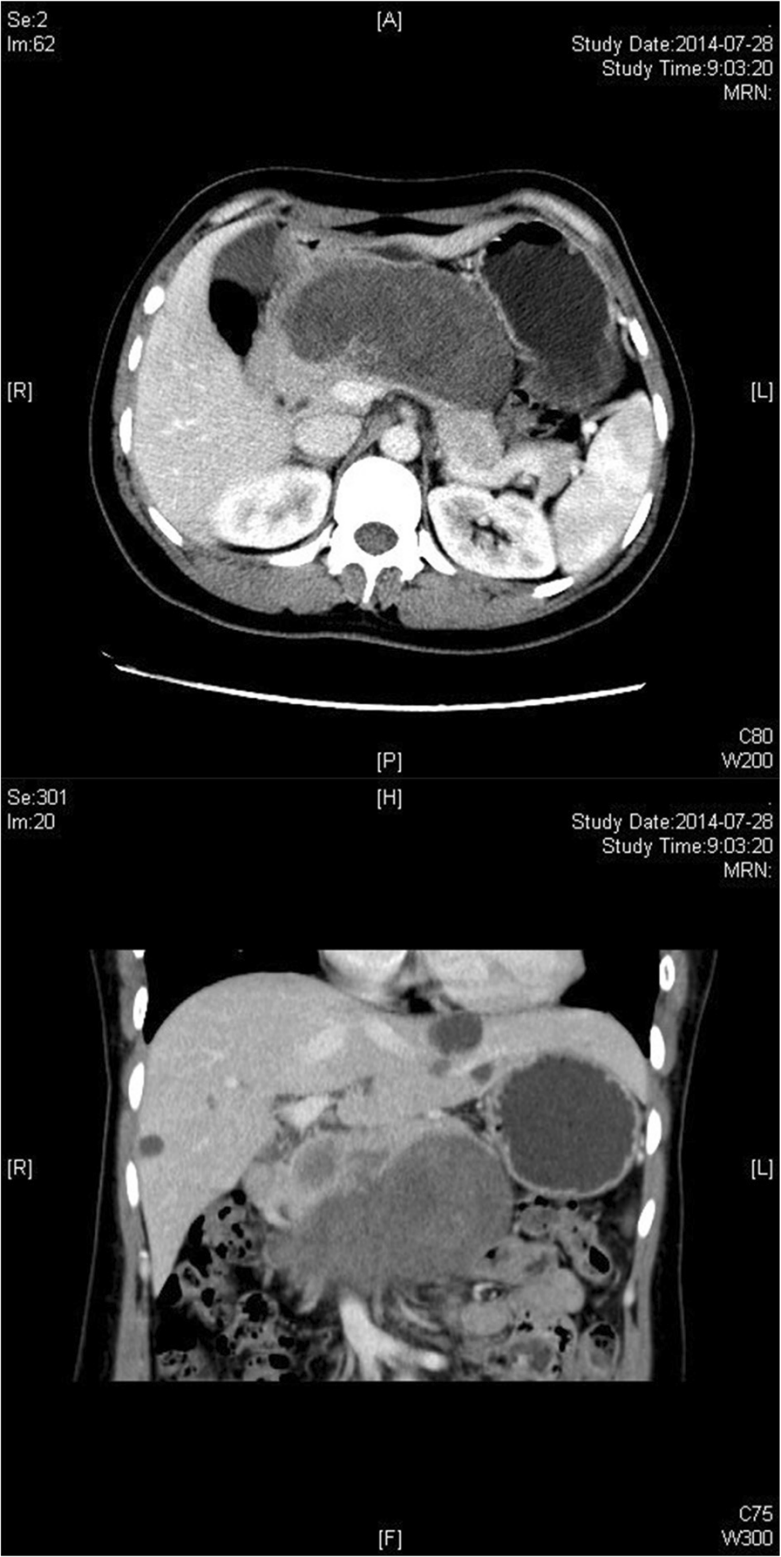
Abdominal dynamic enhanced CT showing a solid and cystic tumor in the body of the pancreas
